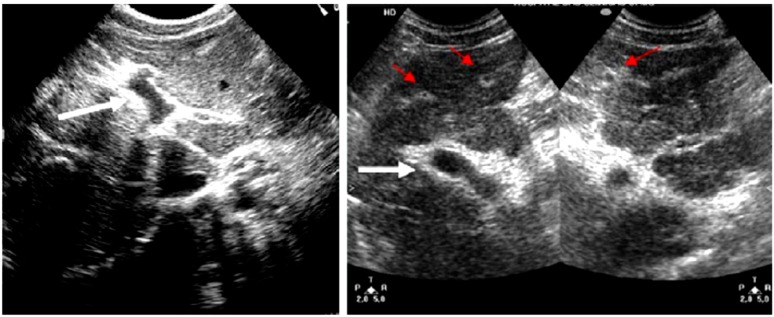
Ultrasound B-scans of the abdomen showing changes caused by schistosomiasis. White arrow: Central periportal fibrosis, red arrows: fibrosis on the periphery of the liver in a patient diagnosed with advanced hepatosplenic schistosomiasis Reproduced with permission [112].
